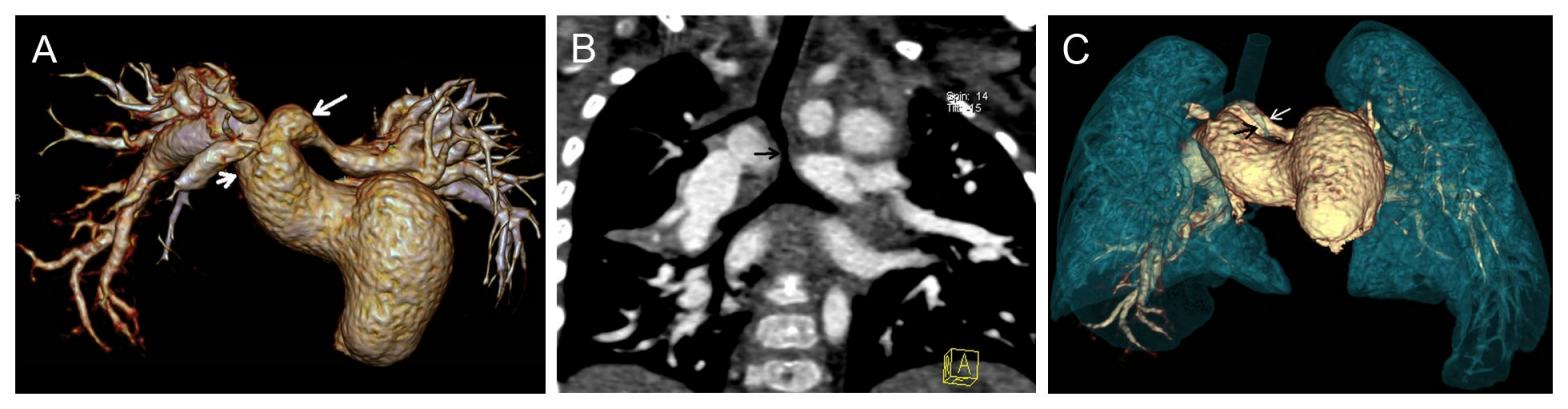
A 1-month girl with congenital heart disease (pulmonary artery sling and ventricular septal defect) was demonstrated the presence of bridge bronchus and bronchial narrowing. A. Volume Rendering (VR) showed the thickening of the pulmonary trunk and the left pulmonary artery (short white arrow) arose from the proximal right pulmonary artery (long white arrow). B. CT coronal image showed the bridge bronchus and bronchial stenosis (black arrow) caused by the anomalous origin of the left pulmonary artery. C. Volume Rendering (VR) of the tracheobronchial tree and the thoracic vascular demonstrated the bridge bronchus and bronchial stenosis (black arrow) due to anomalous origin of the left pulmonary artery (white arrow) compression.
